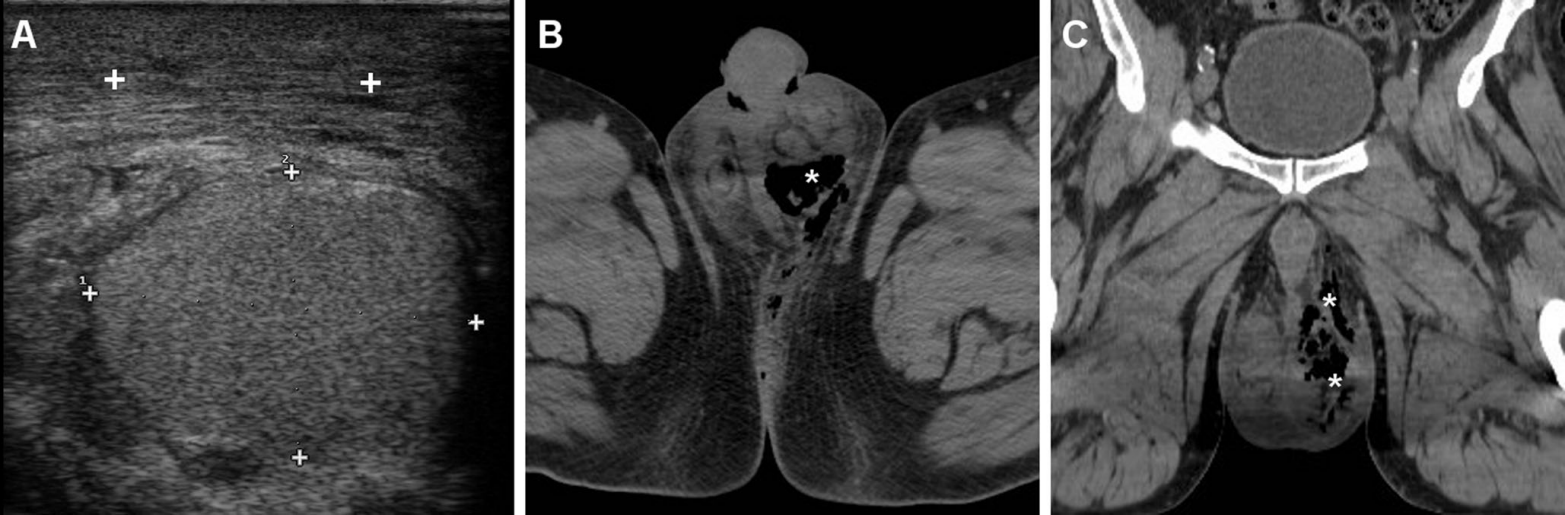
Clinically unsuspected, surgically treated Fournier’s gangrene in a 60-year-old man with diabetes, recurrent UTIs and scrotal swelling. Initial ultrasound (a) depicted prominent inflammatory thickening of the superficial tissues, without hydrocele, and signs of acute orchitis (testis is indicated by calipers). Unenhanced CT (b, c) revealed the presence of intrascrotal gas collections (*)
